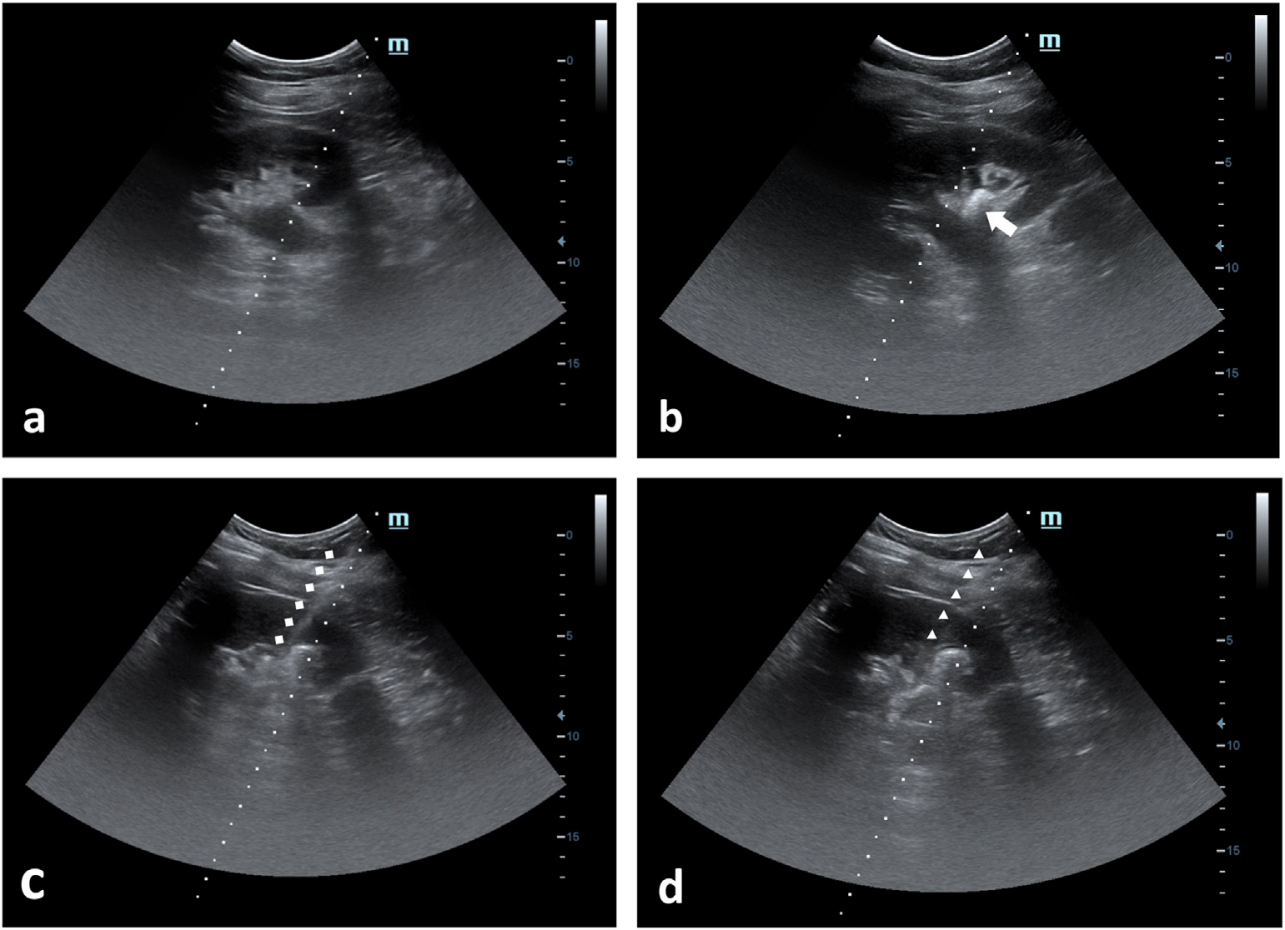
Renal ultrasound in PCNL (a) visualisation of the kidney and its surrounding before ureteral catheter insertion. (b) the tip of the ureteral catheter was seen at the opening of inferior calyx. (c) Needle tract was clearly seen piercing through renal parenchyma, into the pelvic calyceal system. (d) Alken metal dilator can clearly be seen through ultrasound imaging.
